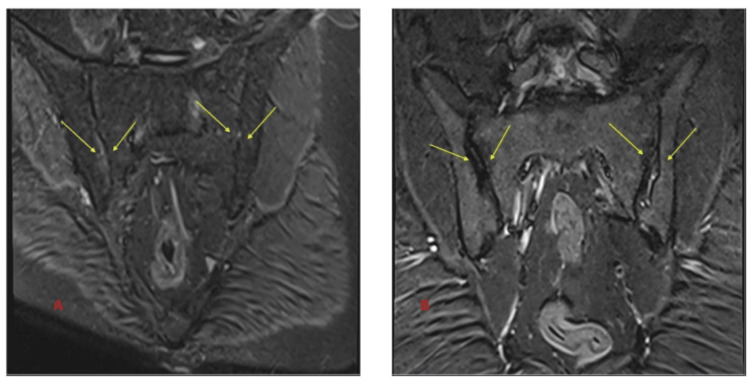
MRI STIR-weighted image of sacroiliac joints before and after treatment (A) The baseline STIR-weighted image highlights significant sacroiliitis with evident joint inflammation, effusion, and structural abnormalities. These findings are consistent with active disease and inflammatory damage prior to treatment initiation. (B) The follow-up STIR-weighted image taken after 12 months of infliximab therapy demonstrates a marked resolution of inflammation and significant improvement in joint integrity, with no new erosive changes observed. MRI: magnetic resonance imaging; STIR: short tau inversion recovery
